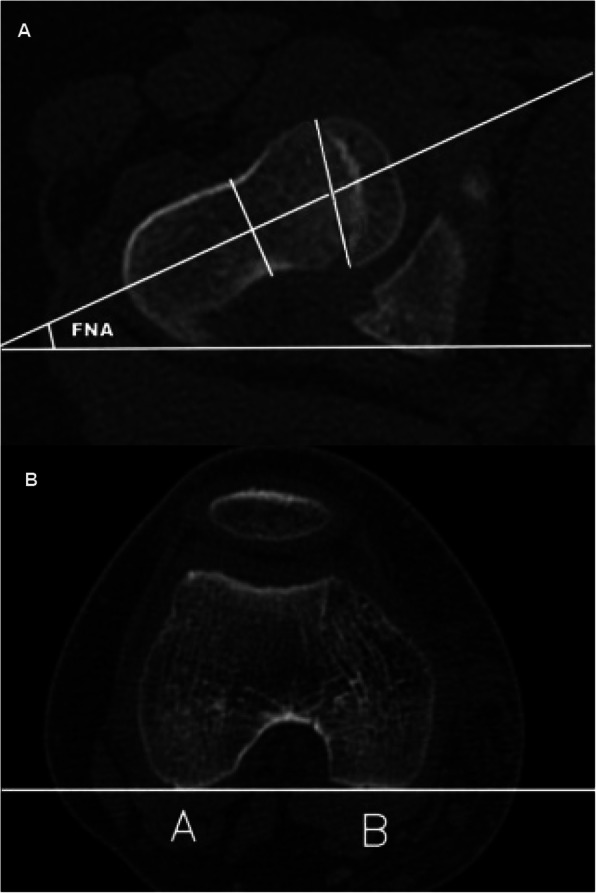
2D-FNA. a CT image of the femoral head and the femoral neck. The center of the femoral head and the femoral neck is connected to determine the femoral head-neck axis. b It shows the largest layer of the two femoral condyles; the points of A and B are the tangent of the posterior rim of the two condyles. The angle between the femoral head-neck axis and the tangent is 2D-FNA
